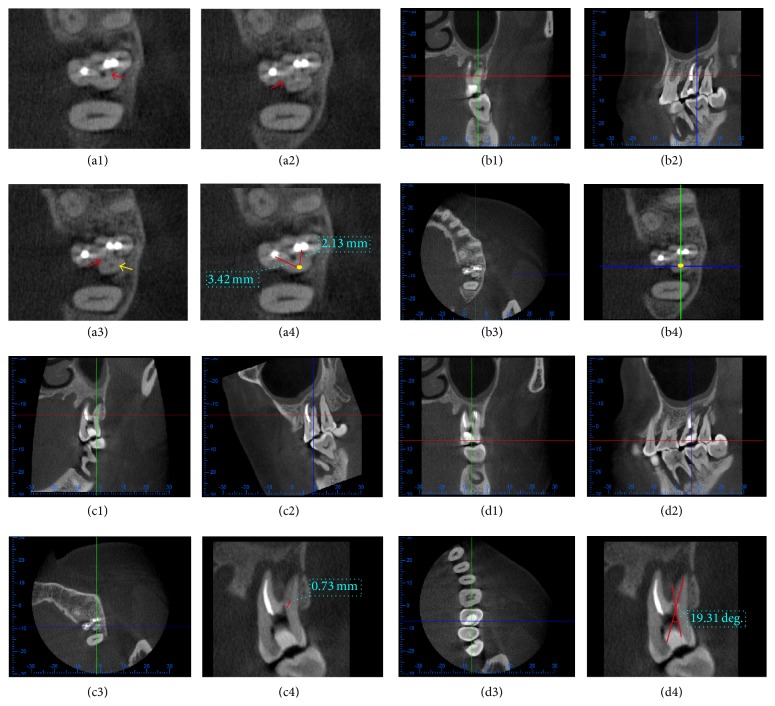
Determination of Parameters. (a1)–(a4) Locate calcified root canal orifice. On cross-sectional plane, canal managed by ultrasonic instrument before CBCT scanning (red arrows) and real canal below calcified orifice (yellow arrow) could be visible. Then, the distance between the calcified orifice and other references (usually orifices of other canals) could be measured to help locate the calcified orifice. (b1)–(b4) Adjust the cross-sectional plane, by moving the red line, to make clear root canal image under the calcification visible. Then, move blue line and green line until their intersection locates on the canal under the calcification, which made the canal appear on buccolingual and mesiodistal planes. (c1)–(c4) Determine the depth of calcification. Rotate the mesiodistal plane to make the blue line in accordance with the same trend of the root canal and overlap the canal resulting in the maximum exposure of root canal on buccolingual plane. Then, the depth of calcification (DOC) in buccolingual direction was determined. In the same way, DOC in mesiodistal direction could be measured (not shown). (d1)–(d4) Determine the direction of calcification. On cross-sectional plane, move the blue line parallel to the mesial or distal wall of pulp chamber (distal wall in this figure) to make the measurement plane in buccolingual direction parallel to distal wall of pulp chamber. Then, the buccolingual instrument angle (BLIA) was determined. In the same way, mesiodistal instrument angle (MDIA) could be measured (not shown).
